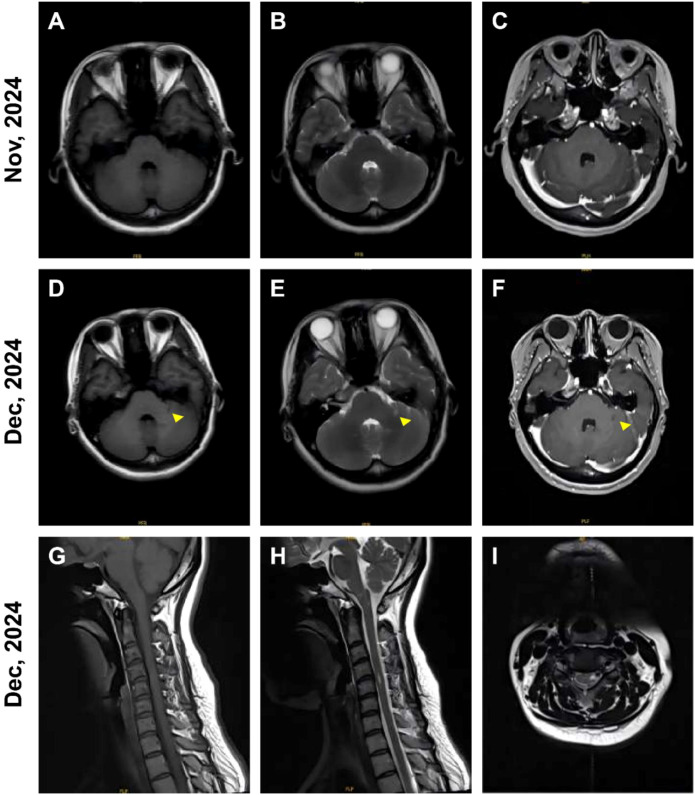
Imaging features of patient 2. (A-C) At the initial admission, brain MRI showed no notable abnormalities. (D-F) At the second admission, brain MRI revealed new lesions in the left cerebellar hemisphere and left middle cerebellar peduncle. (G-I) Cervical spinal cord MRI displayed abnormal signal intensities in the lower medulla oblongata and at the C4-C5 levels.
